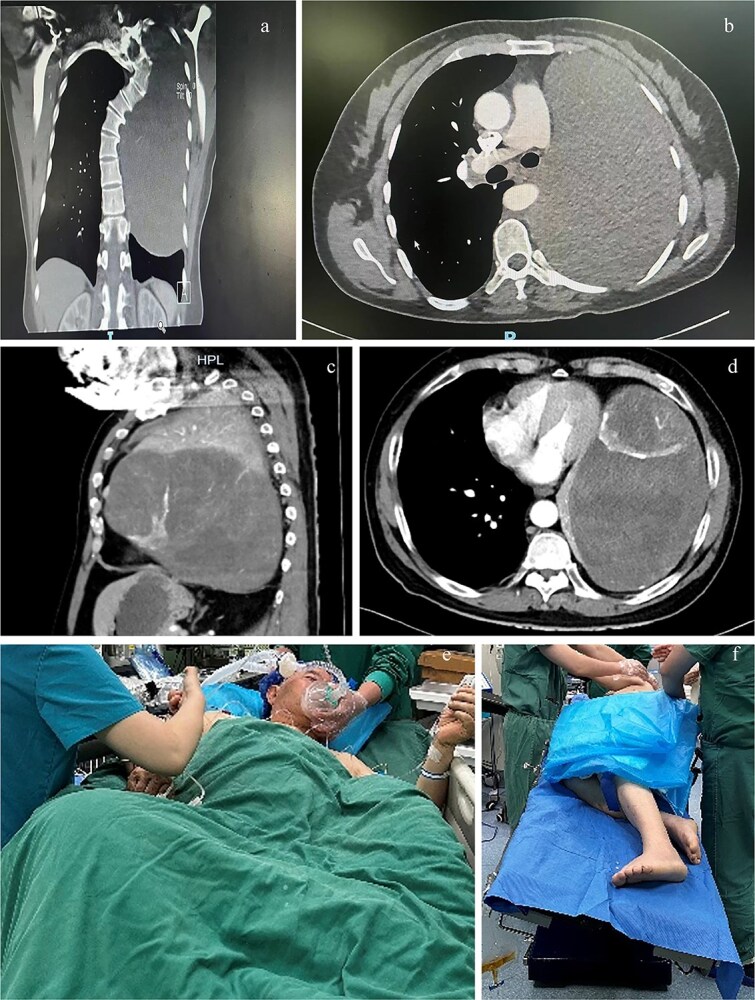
Computed tomography (CT) planes of case 1 in (a) coronal and (b) transverse planes and case 2 in (c) sagittal and (d) transverse planes. The (e) semirecumbent left-tilted posture of case 2 during anesthesia induction and the (f) right lateral decubitus position with the operating bed tilted leftward of case 2 during surgery.
